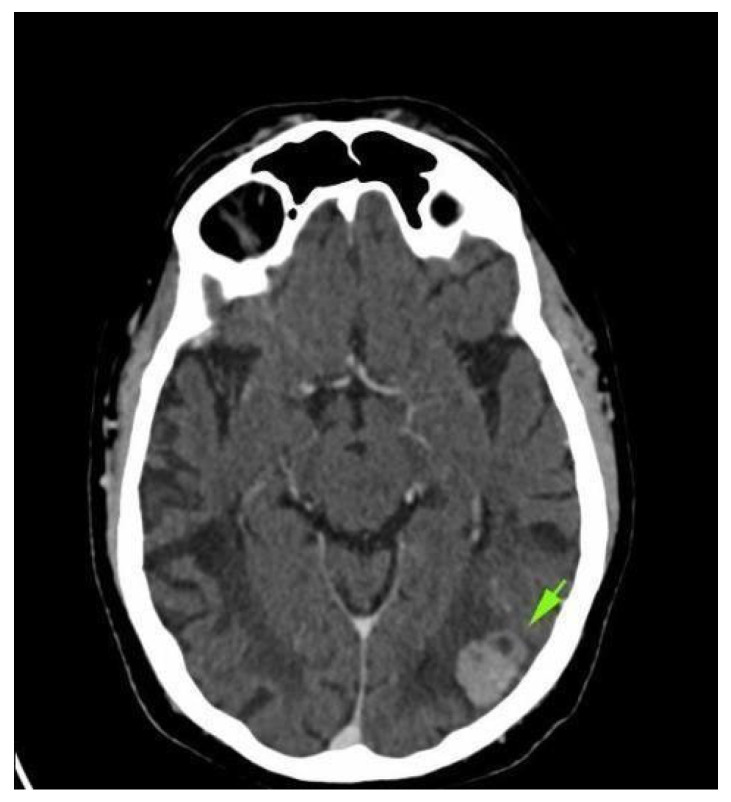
Brain CT scan: left occipital lobe metastases.
